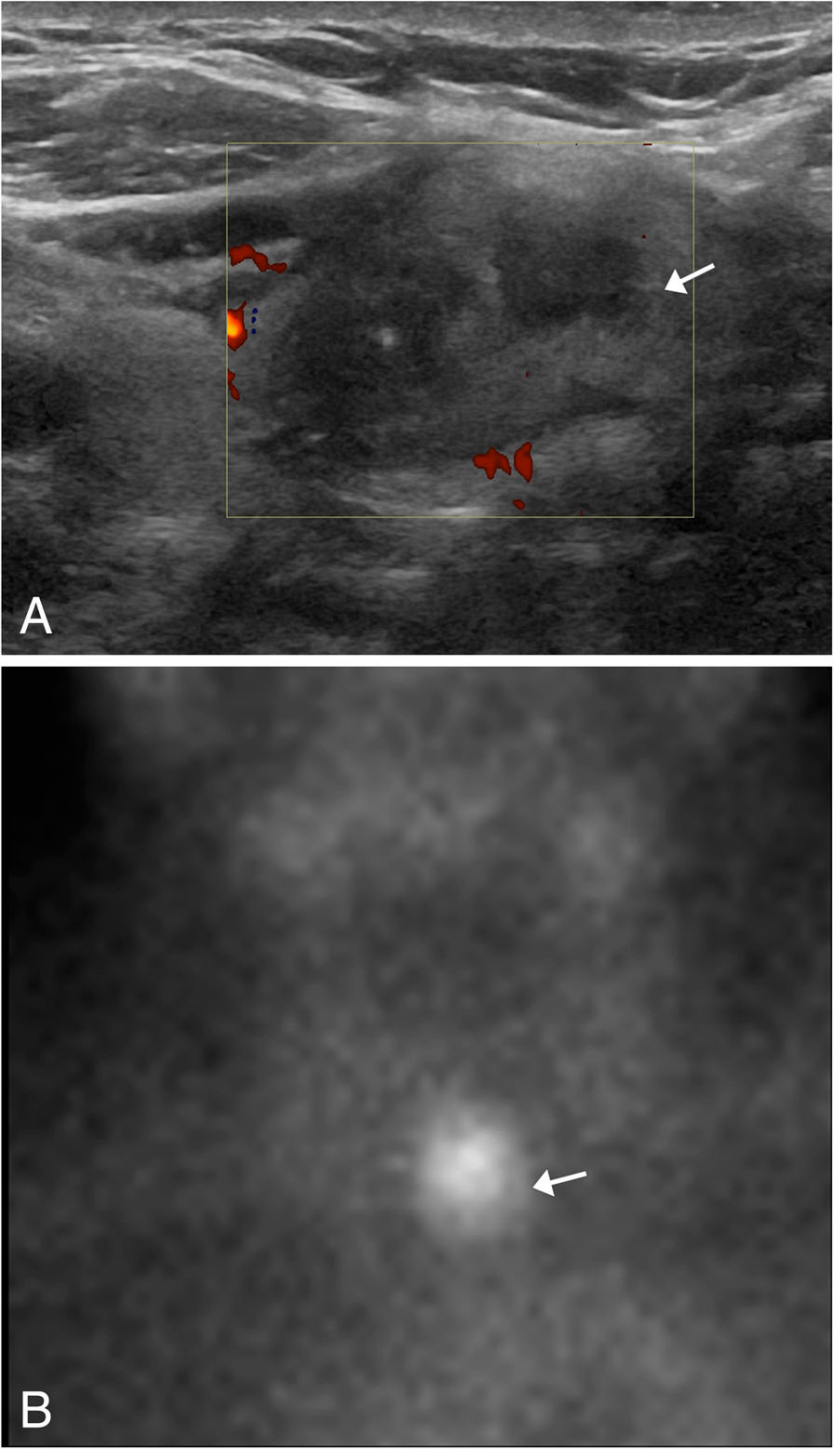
Thyroid adenoma. a Grayscale ultrasound image shows an oval nodule with heterogeneous internal echoes and cystic components without internal flow (arrow). b Iodine 123 thyroid scan shows persistent increased radiotracer uptake within the nodule on the 24-h delayed images (arrow), findings consistent with an hyperactive thyroid adenoma (hot nodule)
